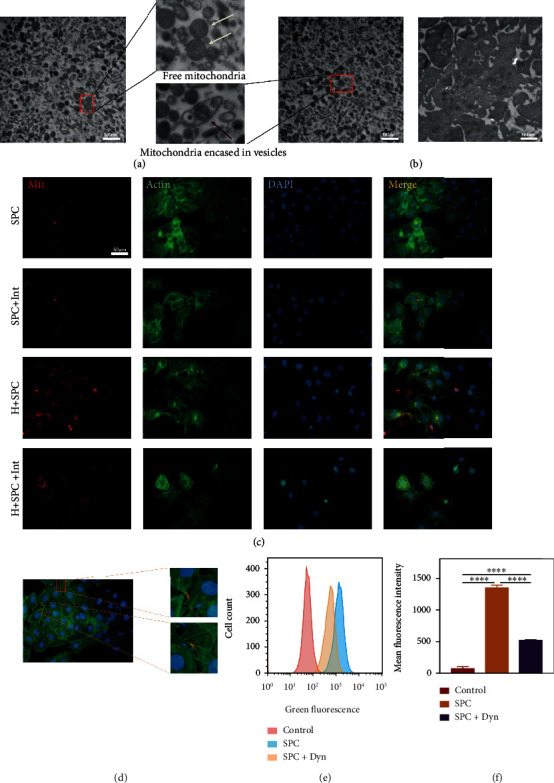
Platelet-derived mitochondria in SPC were taken up by HUVECs. (a) TEM was used to observe the mitochondria released by platelets treated with ultrasound. The white arrow pointed the exposed mitochondria, and the red arrow pointed to the membrane-wrapped mitochondria. (b) TEM was used to observe mitochondria within nonactivated platelets. (c, d) Representative images of HUVECs treated with H_2_O_2_ for 24 h after incubation with SPC or SPC following exposure to mitochondrial inhibitor and previously labeled with Mito Tracker Red. “Mit” labeled the mitochondria. “Actin” labeled the cytoskeleton. “DAPI” labeled the cell nucleus. (e) Flow cytometric analysis of the transfer of platelet-derived mitochondria in SPC to HUVECs previously treated with dynasore, an inhibitor of dynamin-dependent clathrin-mediated endocytosis, and (f) statistical analysis of mean fluorescence intensity. The data in the figures represent the mean ± SD. Significant differences between groups are indicated as ^∗^*p* < 0.05, ^∗∗^*p* < 0.01, ^∗∗∗^*p* < 0.001, ^∗∗∗∗^*p* < 0.0001.
